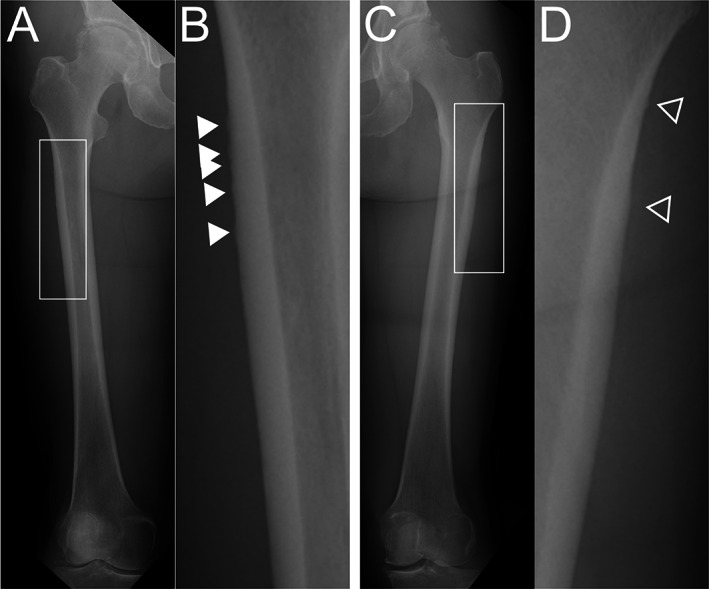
Images for patient with grade 2a radiographic findings. The patient was an 83‐year‐old woman with bone metastasis from lung cancer who had received oncologic denosumab for 65 months. (A) Anteroposterior image of right femur. (B) Enlarged image of white box in Fig 4A showing multiple apparent local thickenings of subtrochanteric lateral cortex (arrowheads, maximum TR 1.2). The multiple thickenings indicated a serrated cortex. (C) Anteroposterior image of left femur. (D) Enlarged image of white box in Fig 4C also shows two apparent local thickenings of subtrochanteric lateral cortex (arrowheads, maximum TR 1.1). She was assessed as grade 2a. TR, thickening ratio.
